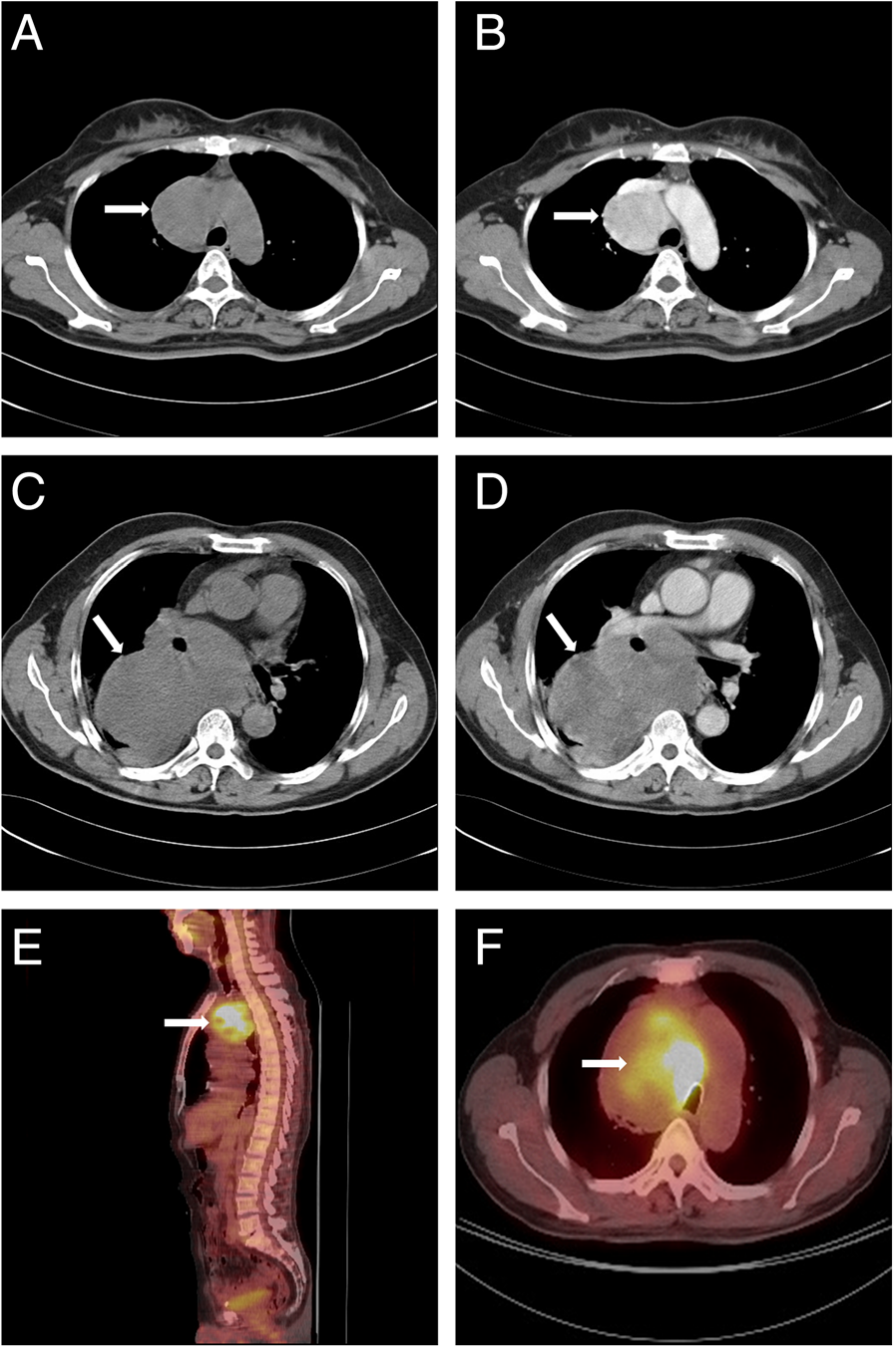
Axial CT of case 1 shows a mass with distinct border in the right upper anterior mediastinum (a), which presents an obviously heterogeneous enrichment after enhancement (b). Axial plain (c) and enhanced (d) CT of case 2 reveal that in the right posterior mediastinum, there is a giant lobulated shadow with moderately heterogeneous enhancement. Sagittal (e) and axial (f) positron emission tomography and CT of case 3 indicate a mass in the middle mediastinum with avid fluorodeoxyglucose uptake (SUVmax = 11.95)
